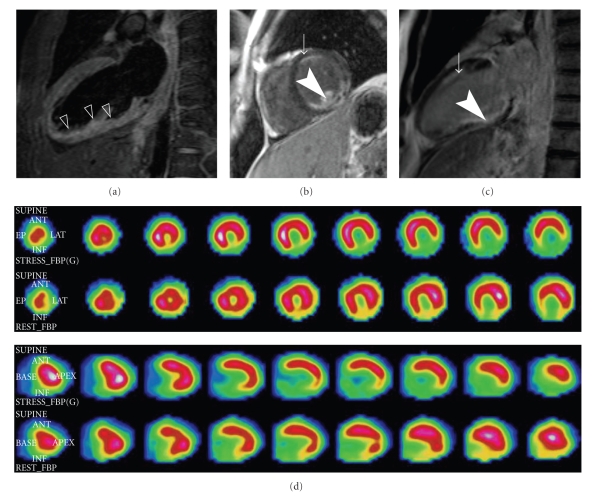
Black blood T2 images (T2 weighted short inversion-time, inversion-recovery (STIR) breath hold pulse sequences) suggested myocardial oedema (arrowheads) strictly in the inferior wall (Figure 2, Panel (e)). Gadolinium-enhanced images (Inversion Recovery turboFLASH 3D short axis and long axis sequences) demonstrated transmural late enhancement of the inferior wall (arrowheads) associated with late microvascular obstruction (Panel (f) and (g)). Furthermore, these sequences showed a subendocardial delayed enhancement area (arrows) which was located in the anterior wall at mid-ventricular level in favour of a limited necrosis (Panel (f) and (g)). Myocardial perfusion scintigraphy with thallium-201 suggested hypoperfusion in the inferior and lateral walls during stress but did not detect at rest any hypouptake in the anterior wall (Panel (h)).
